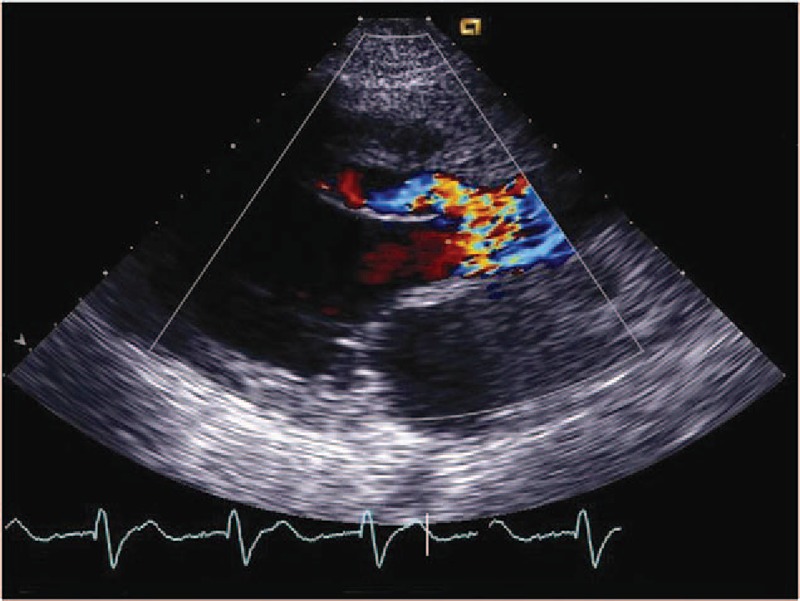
(Patient #2) Transthoracic echocardiogram (parasternal long axis view with color) showing the communication between the right sinus of Valsalva aneurysm and the dissection in the interventricular septum.
